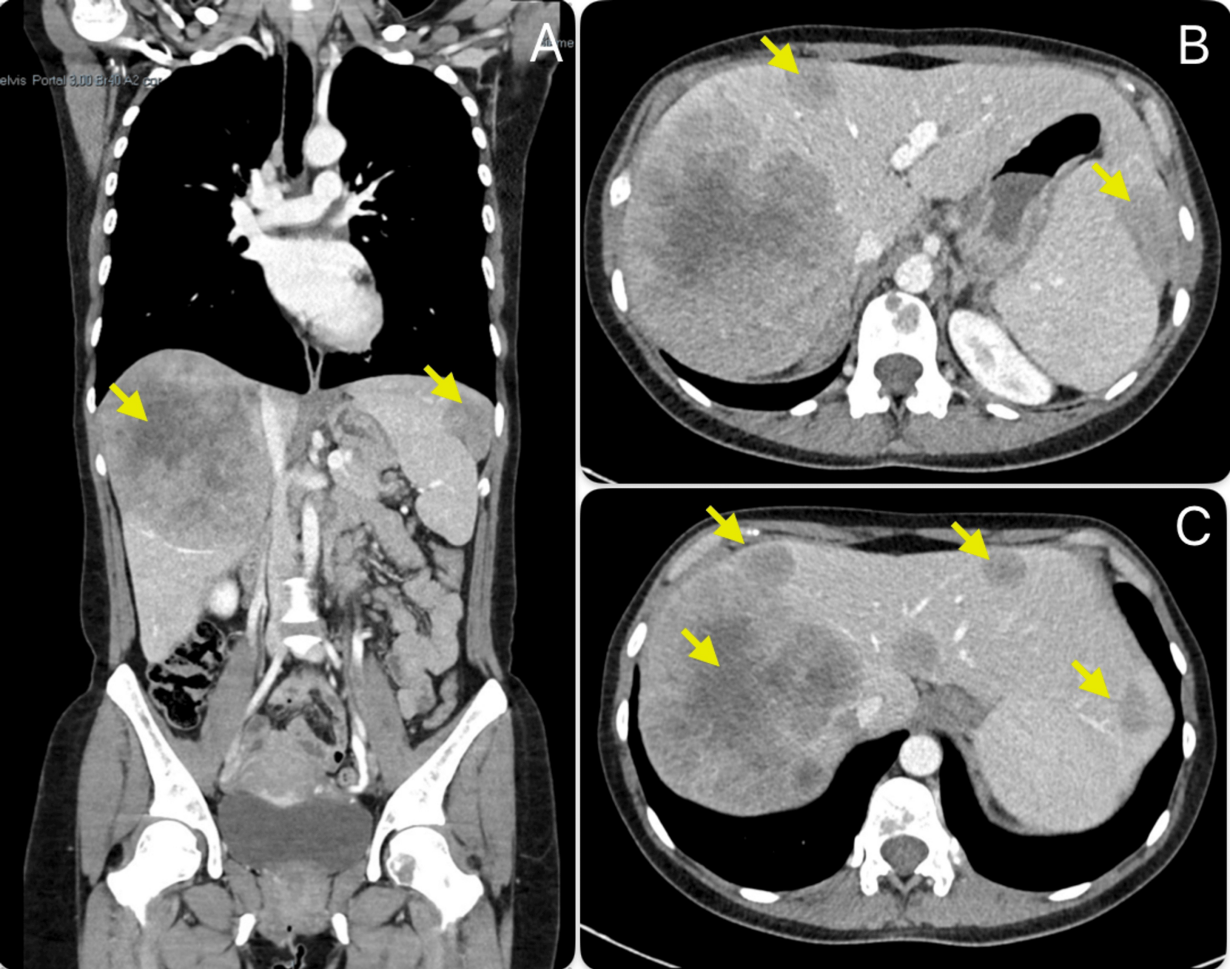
Full body computed tomography scan CT scan showing several hepatic metastases and a splenic metastasis (coronal section of abdominal CT scan - A; transverse section of abdominal CT scan - B, C).
